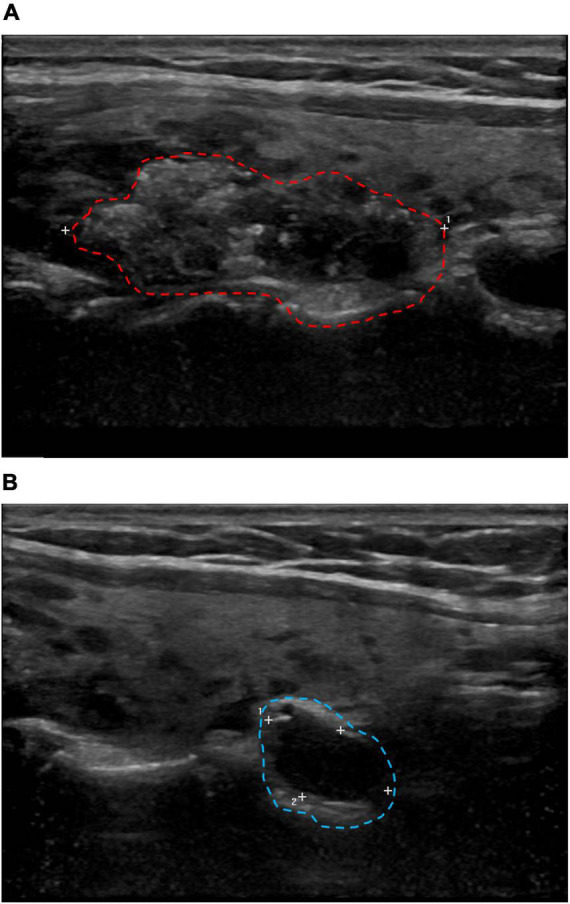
Cervical B-ultrasound. (A) Solid mass in the middle and upper pole of the right lobe of the thyroid gland, 33.4 × 14.5 × 21.2 mm (red circle). (B) The right cervical region VI lymph node showed enlargement, up to 12 × 6 mm (blue circle).
